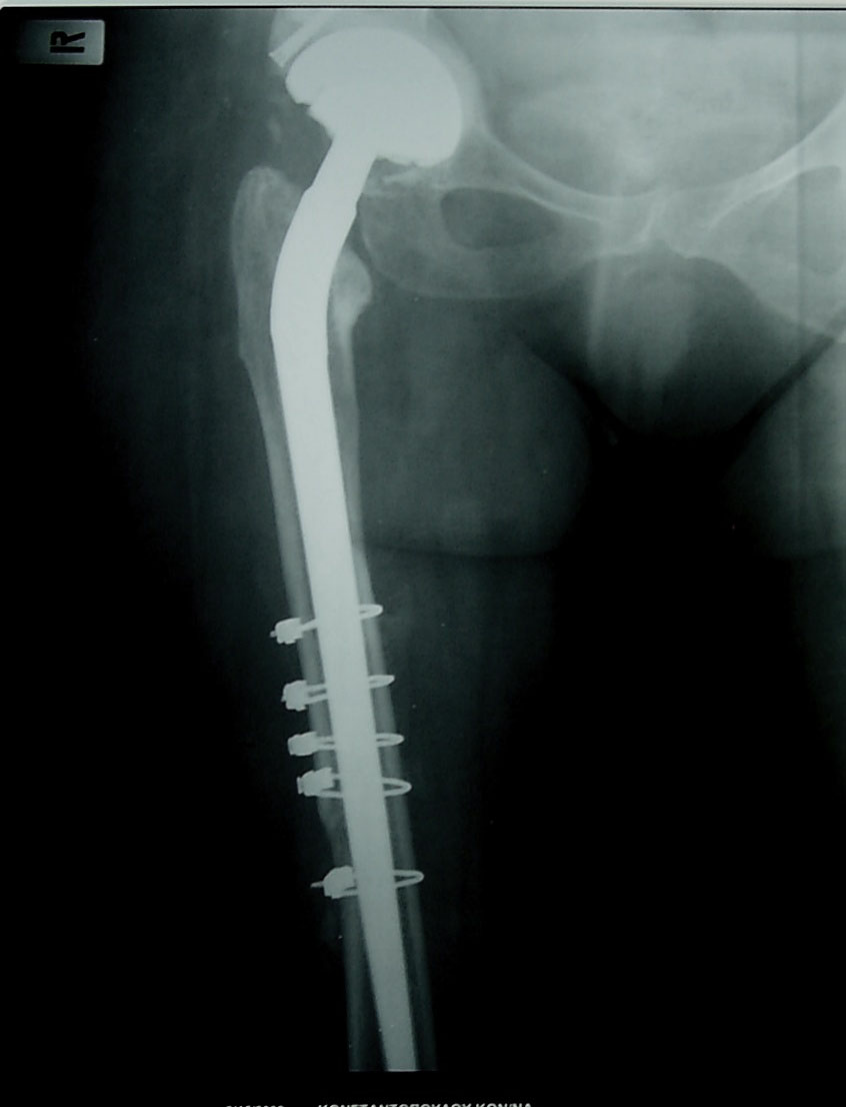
Anteroposterior X-ray at three postoperative months. Fracture has healed.
